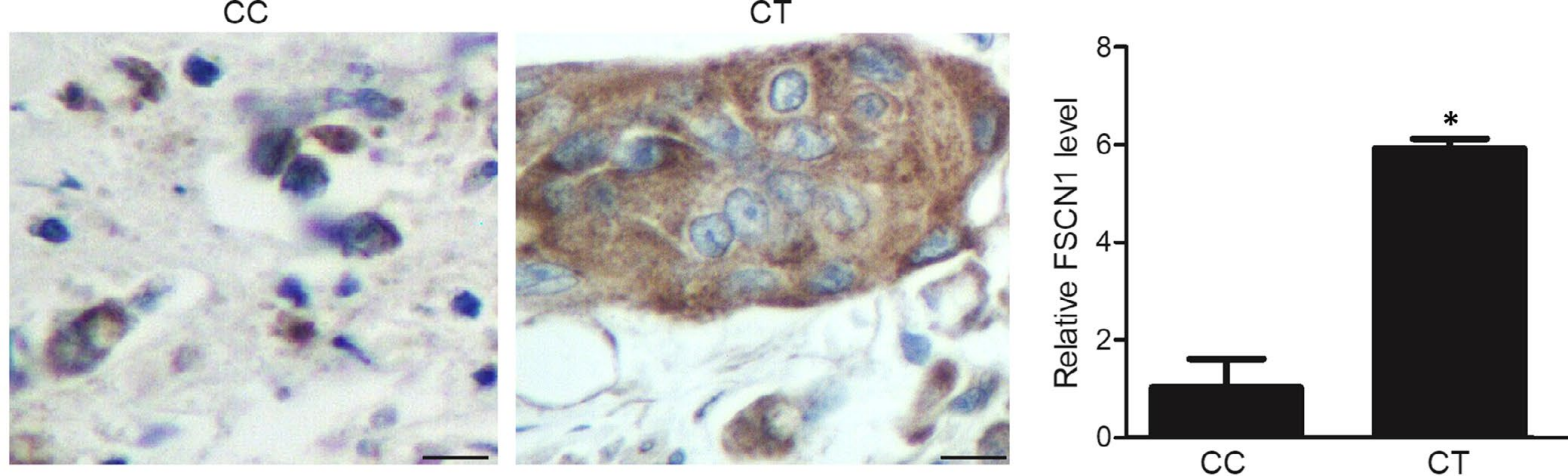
IHC assay indicated the expression of FSCN1 protein in colon cancer patients with CC and CT genotypes (^*^
